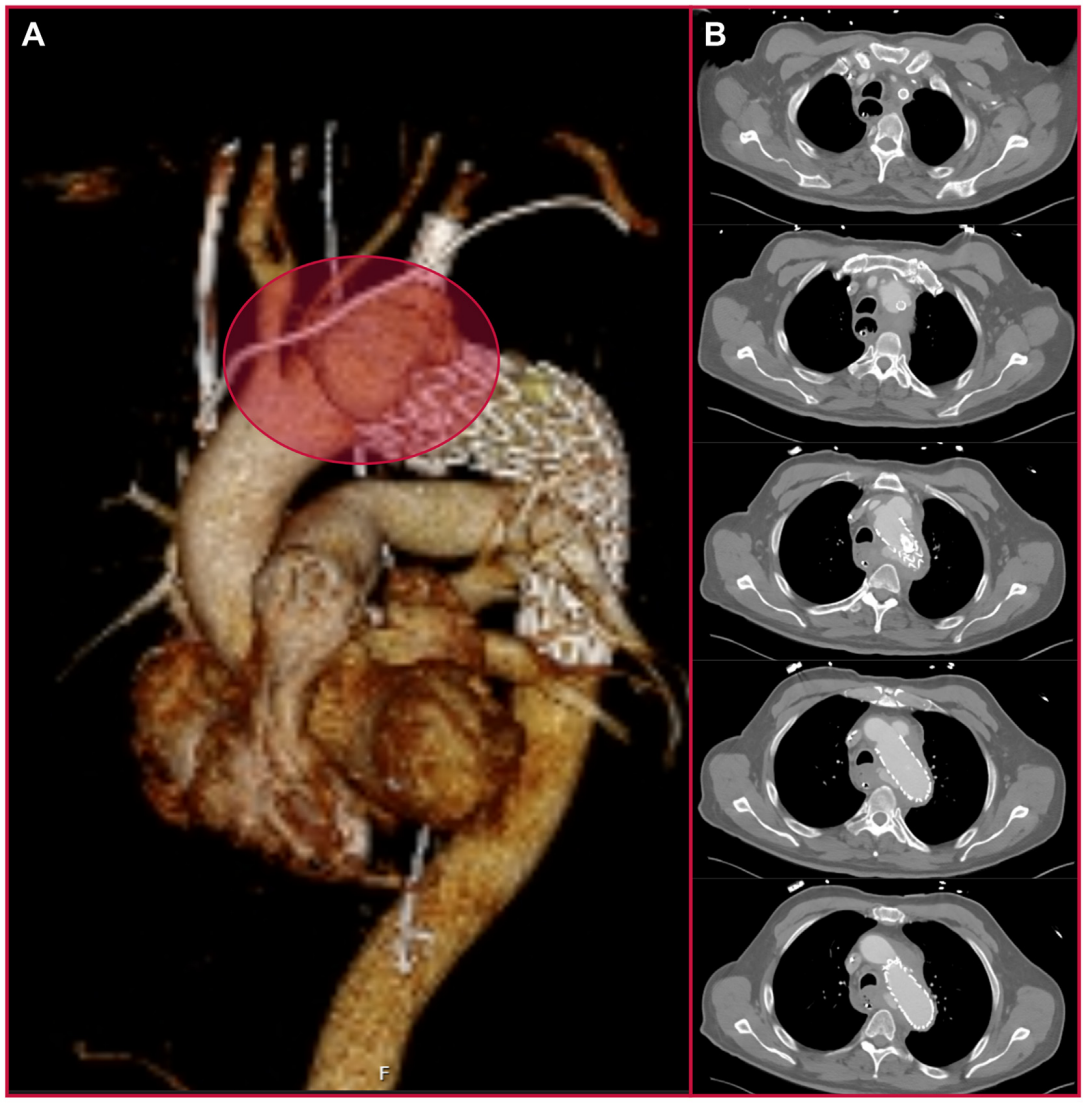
Final computed tomography (CT) scan revealing new anterolateral pseudoaneurysm with recurrent filling of original posteromedial aortic defect. (A) Three-dimentional reconstruction anterior view. (B) Axial CT angiography series.
